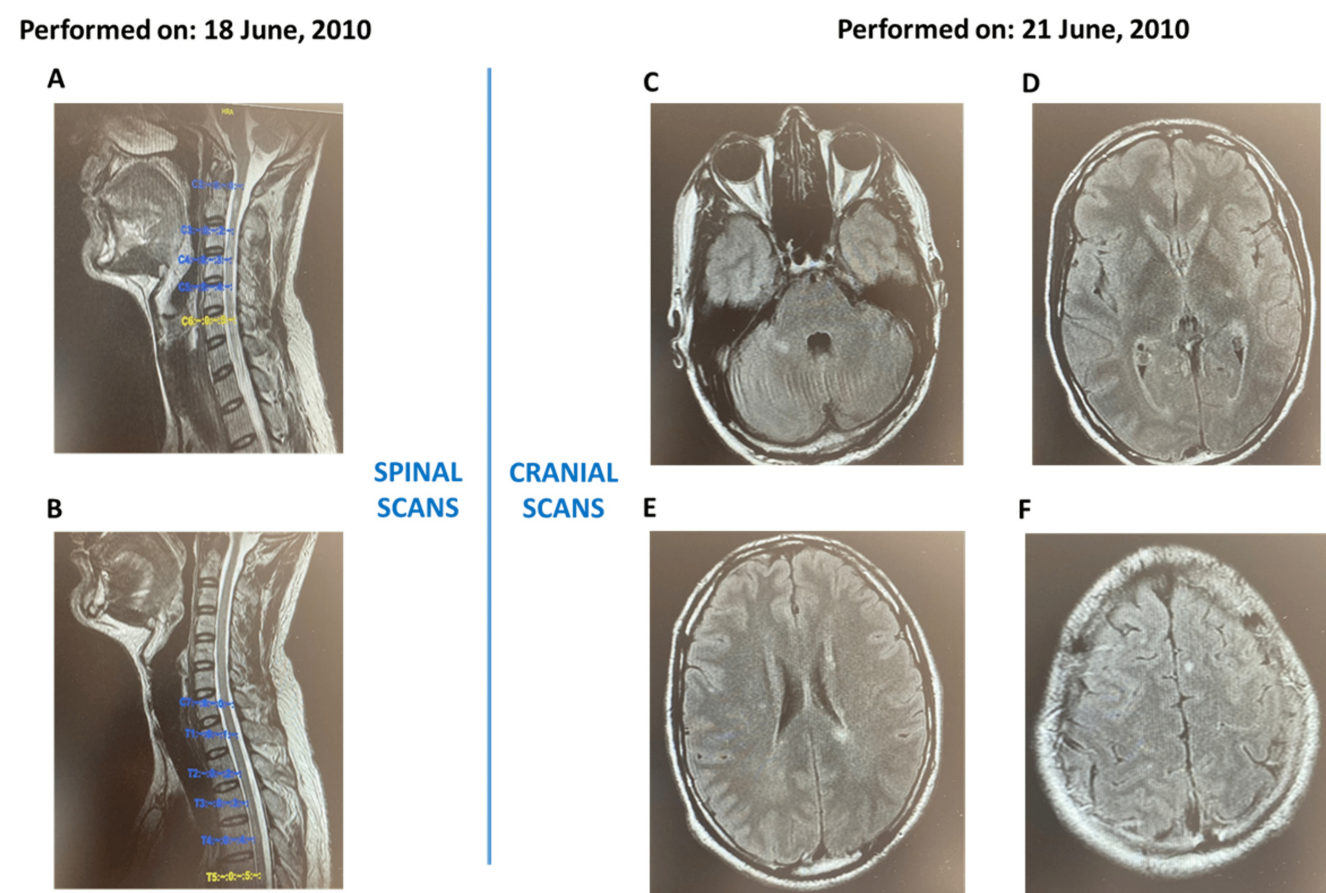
(A-F) Initial magnetic resonance imaging scans for Case 2
